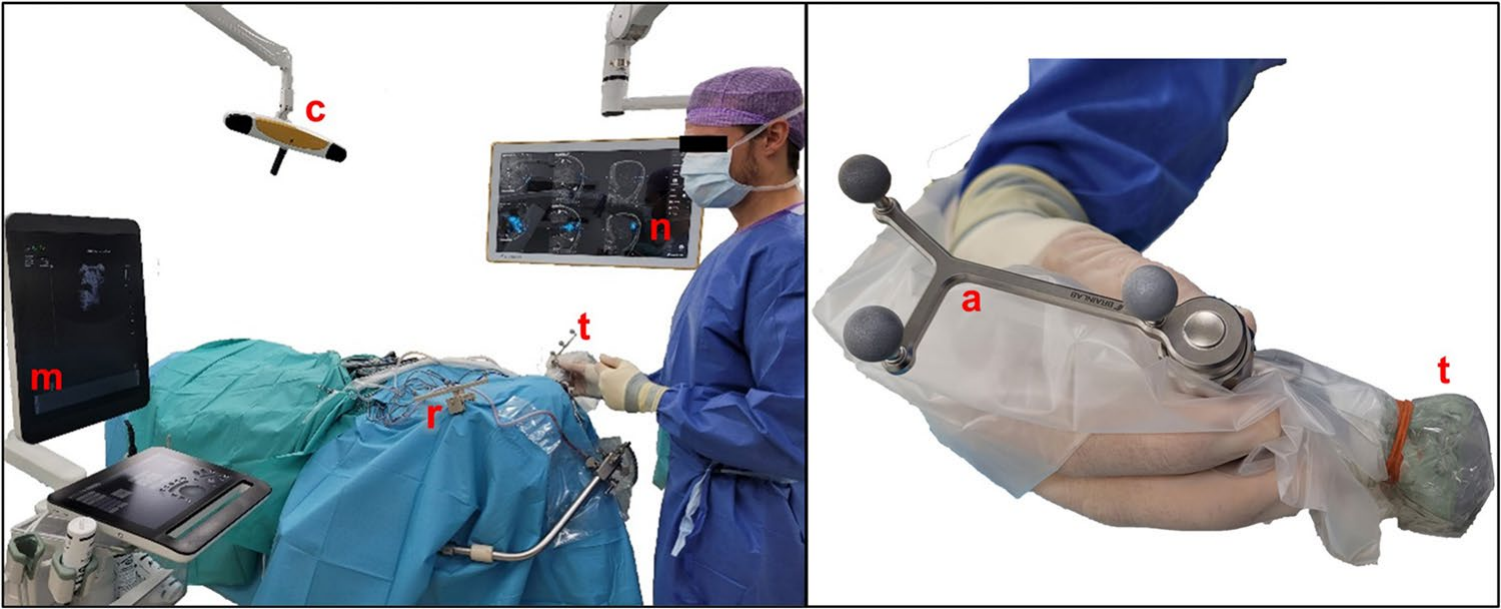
Navigated intraoperative acquisition set-up. Left image: set-up during iUS acquisition. Reference array (r) and transducer (t) are both optically tracked by stereotactic camera (c). The live ultrasound image is shown on the ultrasound machine (m) and the navigated image is overlaid on MRI (n). Right image: N13C5 transducer (BK5000, BK Medical, Denmark) (t), draped in a sterile cover with the sterile array (a) for optical navigation is attached (Brainlab, Munich, Germany)
